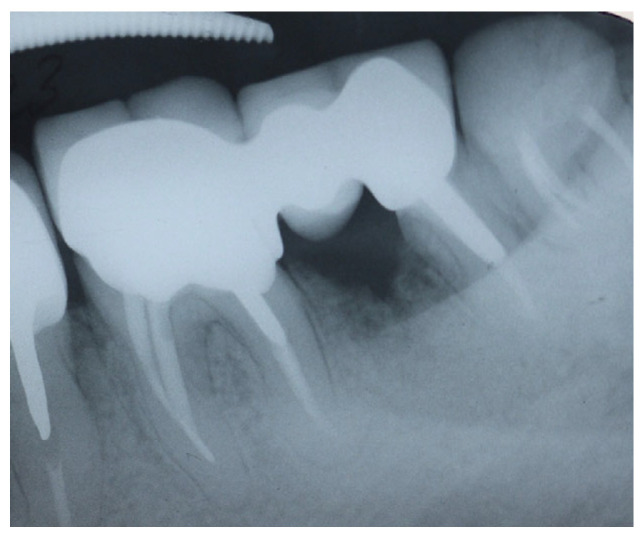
X-ray at 1-year recall, after restoration. An ongoing bone formation on the extraction socket is evident.
